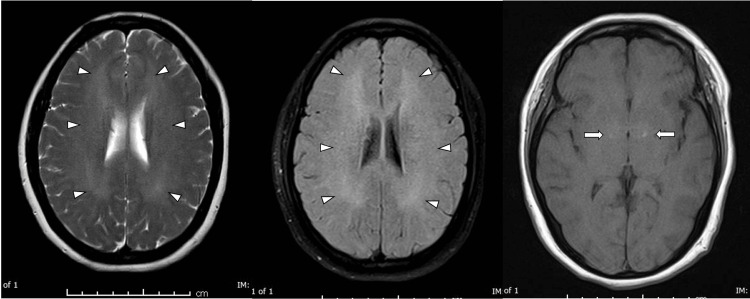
MRI of the brain showing mild diffuse T2 and FLAIR hyperintensity affecting the white matter (arrowheads). Subtle T1 hyperintensity is seen in the globus pallidus bilaterally (arrows). MRI, magnetic resonance imaging; FLAIR, fluid-attenuated inversion recovery
